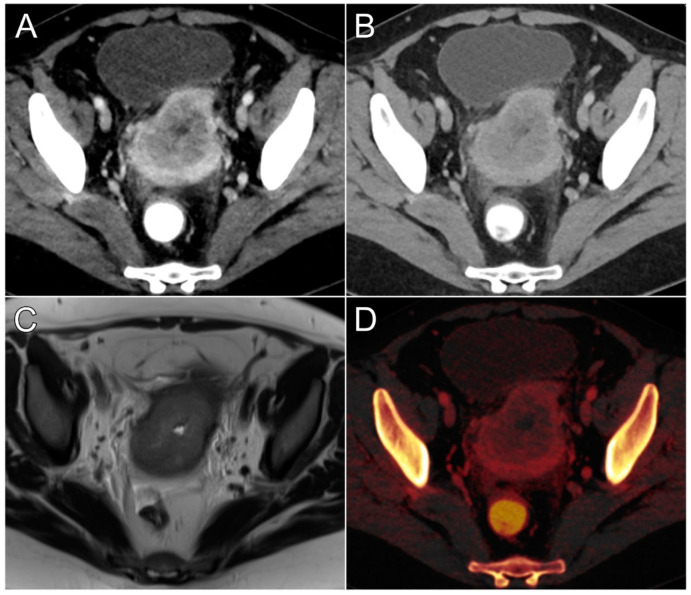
Primary staging of endometroid adenocarcinoma in a 53-year-old woman with improved assessability of myometrial invasion in a virtual monoenergetic reconstruction at 40 keV (A) compared to polyenergetic imaging (B). Tumor delineation in 40 keV images is even comparable to T2-weighted MRI (C) in this case. The additionally reconstructed iodine map (D) allows for quantitative assessment of iodine concentrations in tumors and healthy myometrial tissue.
